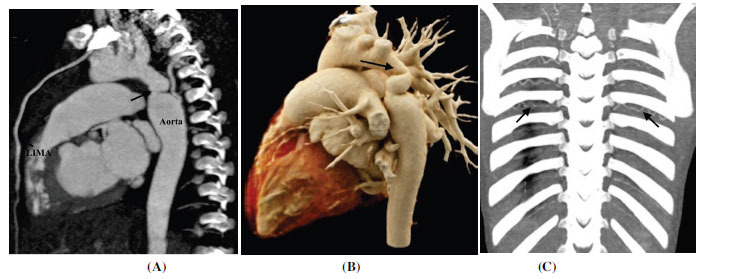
Post ductal aortic coarctation. (A) Sagittal contrast-enhanced computed tomographic angiography image showing focal narrowing of the aortic lumen (arrow) distal to the origin of the left subclavian artery. The left internal mammary artery is enlarged to provide collateral circulation to descending thoracic aorta (B) Volume rendered technique image confirms the findings. (C) Sagittal and (E) Coronal contrast-enhanced maximum intensity projection images showing collateral circulation (arrows) through enlarged left internal mammary retry and intercostal arteries respectively. Abbreviation: LIMA; left internal mammary artery.
